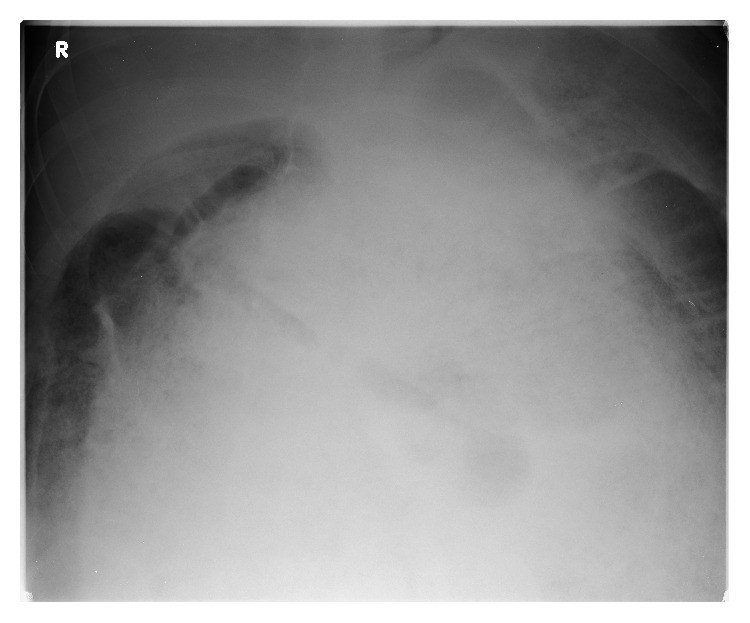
Plain preoperative erect chest radiograph.
